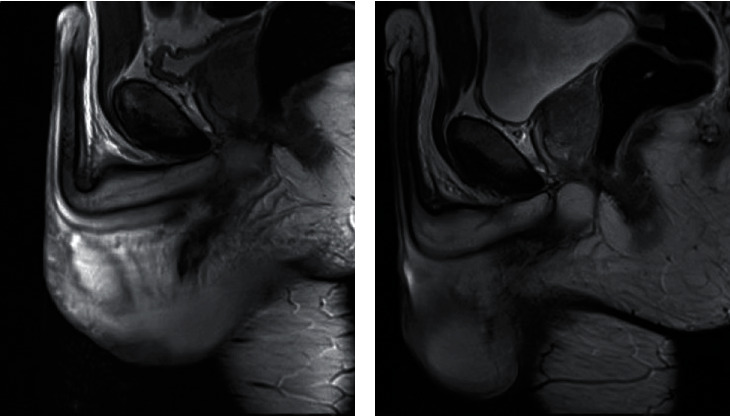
T2-weighted sagittal pelvis MRI; (a) hematoma at the base of the scrotum, (b) complete resolution at one month.
